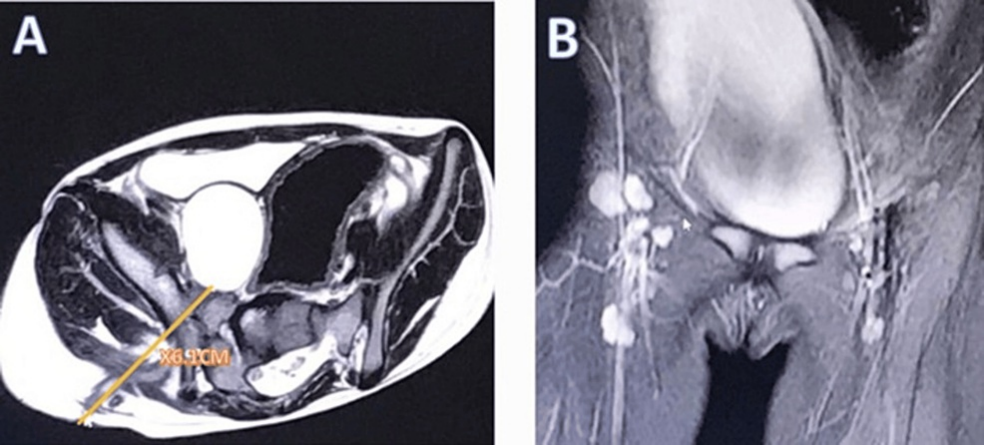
Magnetic resonance imaging (MRI): (A) Axial cross-section shows a large enhancing right gluteal collection with a deep fistula, the tract is about 6.1 cm long, indicating the described cystic lesion and both hips were normal; (B) the coronal cross-section shows the elongated cyst.
